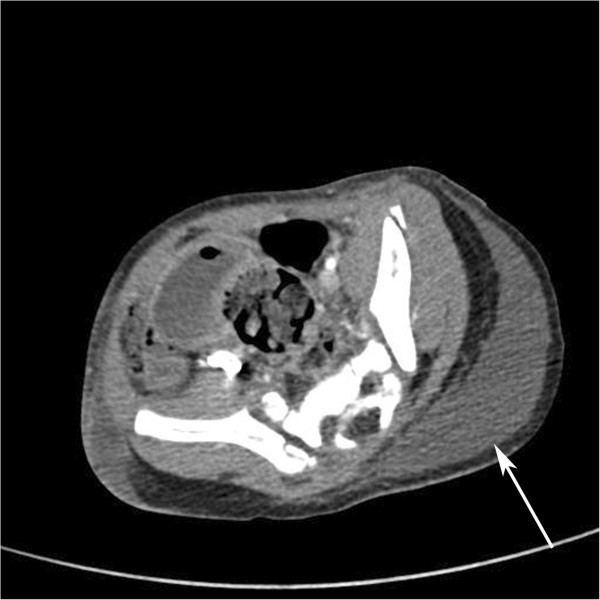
Contrast-enhanced axial CT scan obtained on day 9. The scan shows a well-defined isodense to hypodense fluid collection (arrows).
